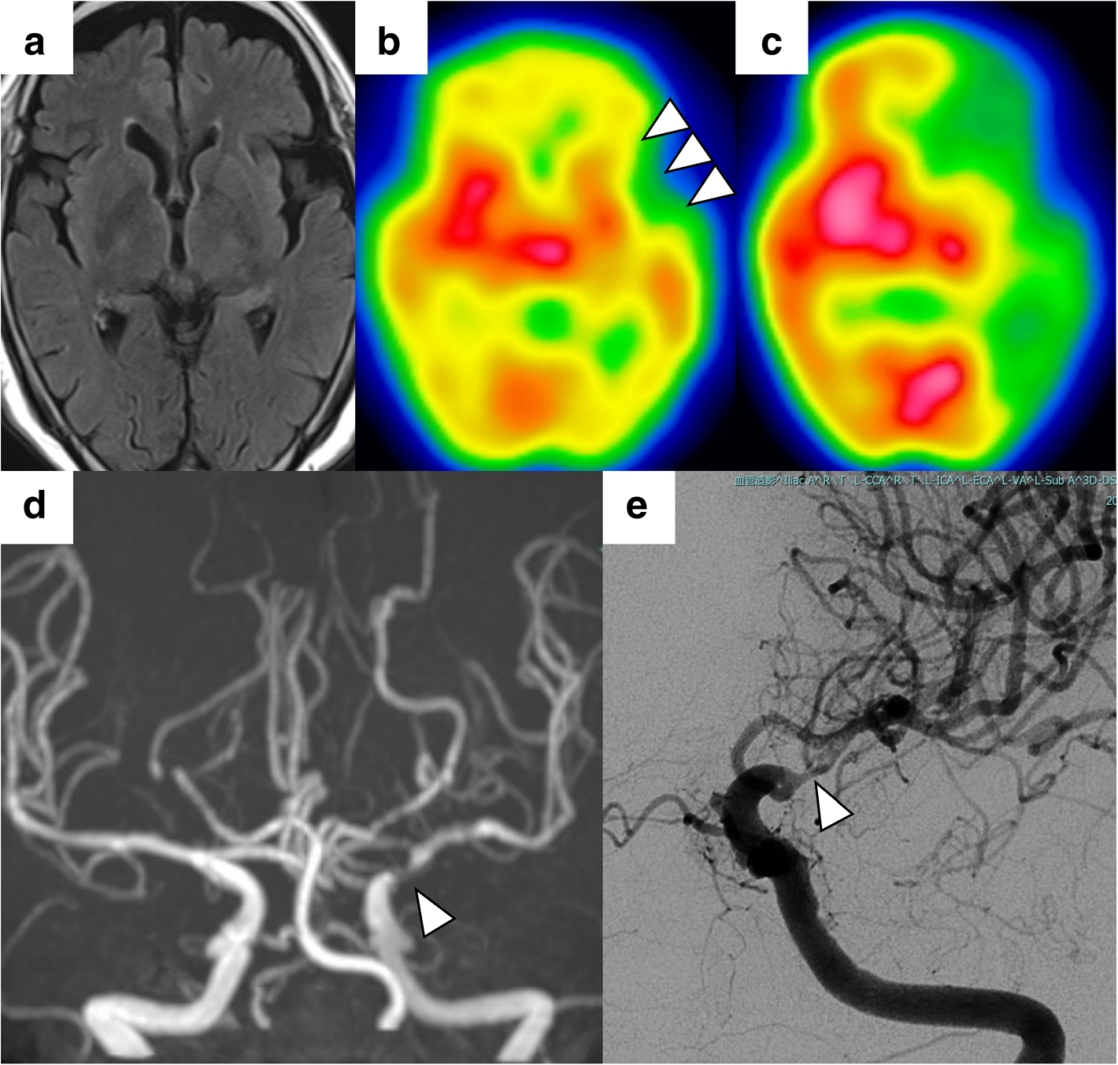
Hemichorea developed in a case of *RNF213*-related vasculopathy showing no ischemic stroke on an MRI fluid-attenuated inversion recovery image (a) but reduced cerebral blood flow in a resting state (b, arrowheads) and after acetazolamide challenge (c) of ^123^I-iodoamphetamine-single photon emission computed tomography with unilateral intracranial stenosis of the left internal carotid artery on MRA (d) and digital subtraction angiography (e, arrowhead)
